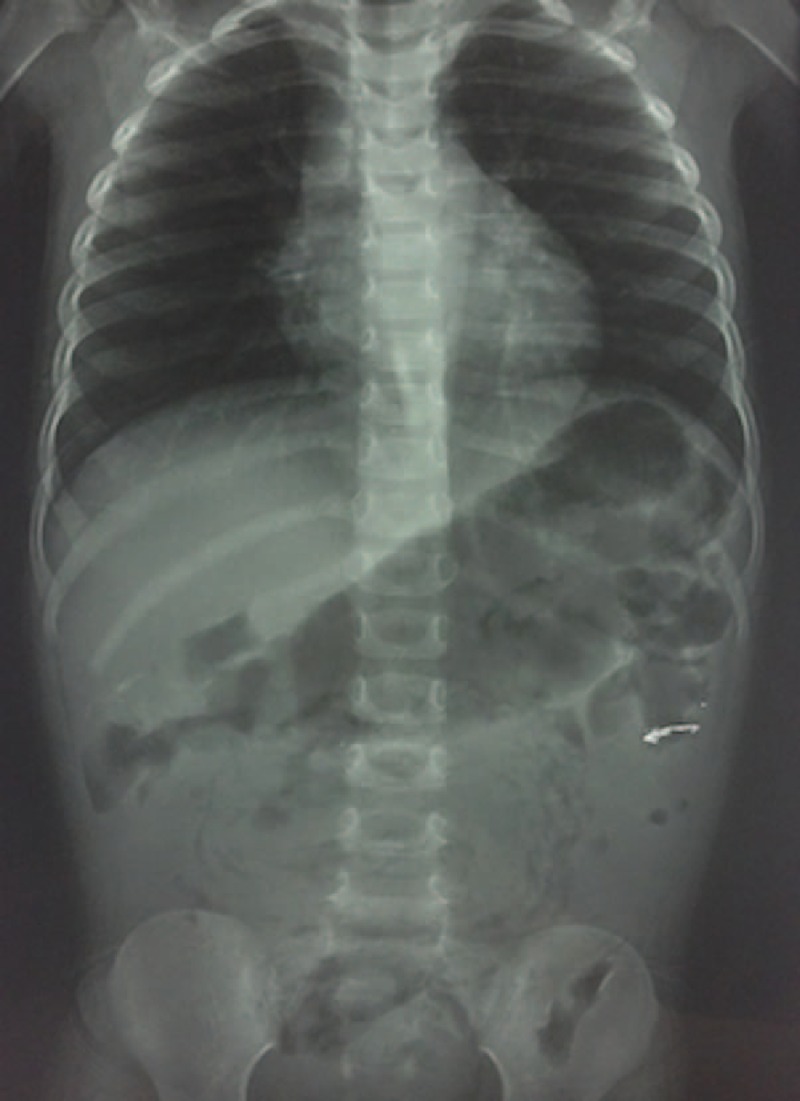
Abdominal radiography presenting multiple air-fluid levels, intestinal loops dilated, and a whirlpool image in the inferior hemiabdomen.
